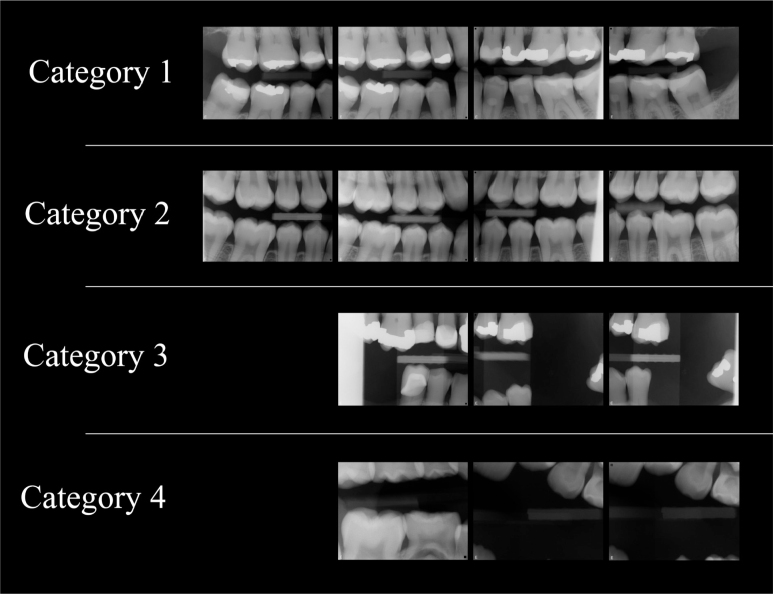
Examples of bitewing examinations of different categories based on how well they fulfilled the quality requirements for caries and marginal bone level diagnostics. Category 1: All required interproximal surfaces depicted with minimal or no overlap, including all required areas of the marginal bone. Category 2: Marginal bone levels insufficiently depicted posterior to the most posterior teeth in all required areas, but otherwise healthy radiographic dental conditions. Category 3: Marginal bone levels insufficiently depicted in quadrants 1, 2, and 3, interproximal surfaces are missing or overlapped, including insufficient biting on the sensor holder. Category 4: Requirements not fulfilled and complete retake needed.
